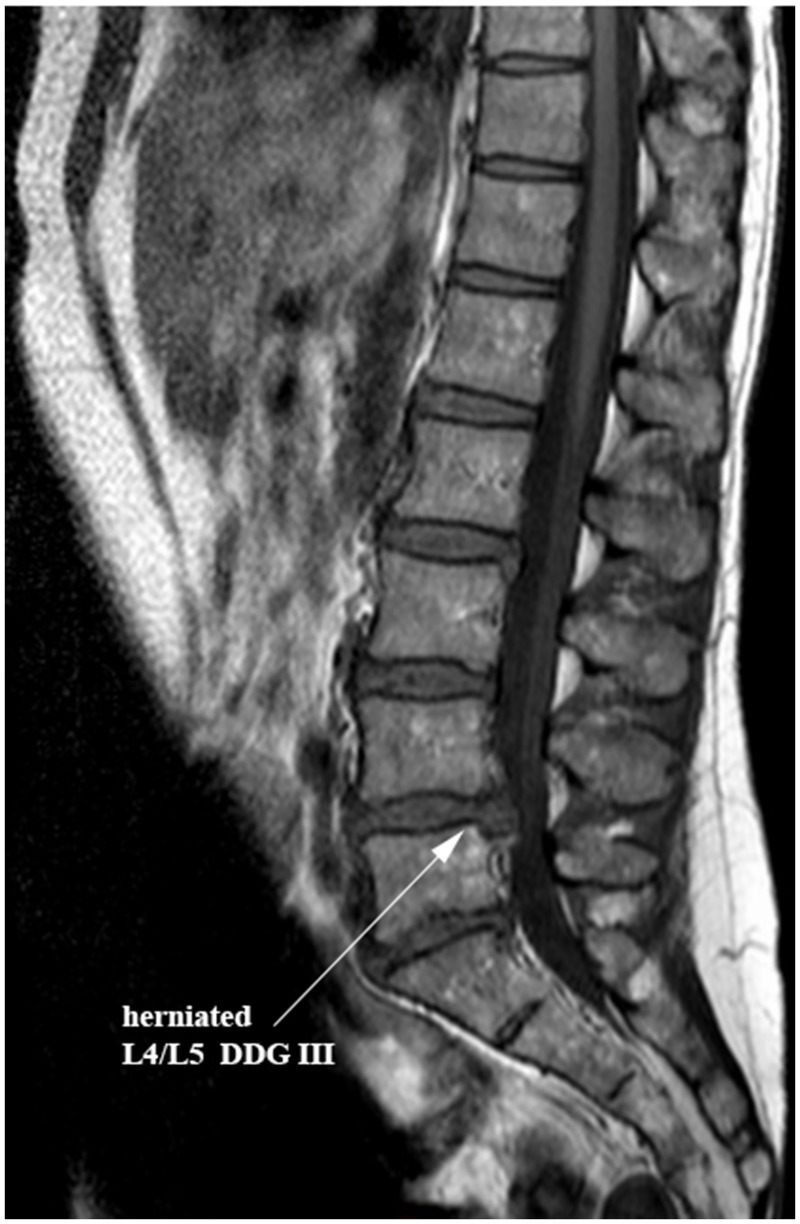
A representative MRI picture of the IVD samples. 12 patients with lumbar discs of degeneration grade III (mean age of 49 years: range 34–61 years) were involved in this study. A representative MRI exhibits a herniated lumbar disc (L4/L5) of degeneration grade III (DDG III) with nerve root compression that remained unresponsive to non-operative treatment for six weeks.
